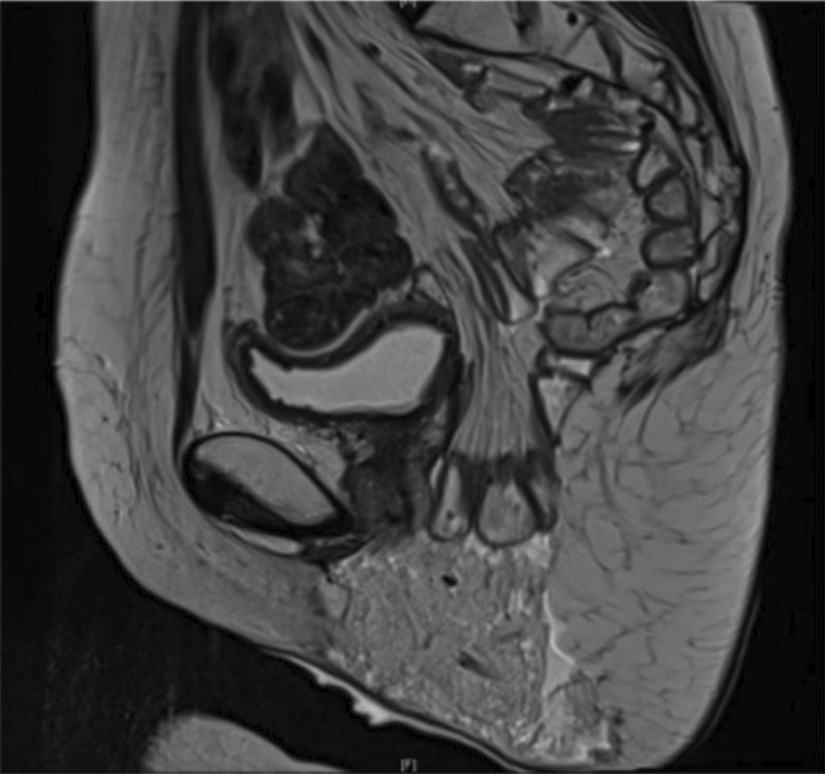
Sagittal pelvic MRI of the perineal hernia
